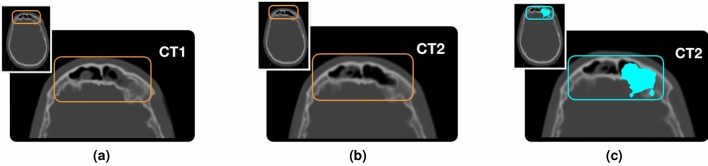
Left: view of CT1 of PZC. Center: view of CT2 of PZC. In both images the region interested by the morphological change, spotted via the re-evaluation CT, is highlighted in orange. The emptying of the region can be clearly seen. Right: CT2 of PZC with superimposed, in cyan, the distribution of the PCAs belonging to the SPBs that have a p-value below 0.02 when testing the consistency between the first monitored fraction and the last one.
